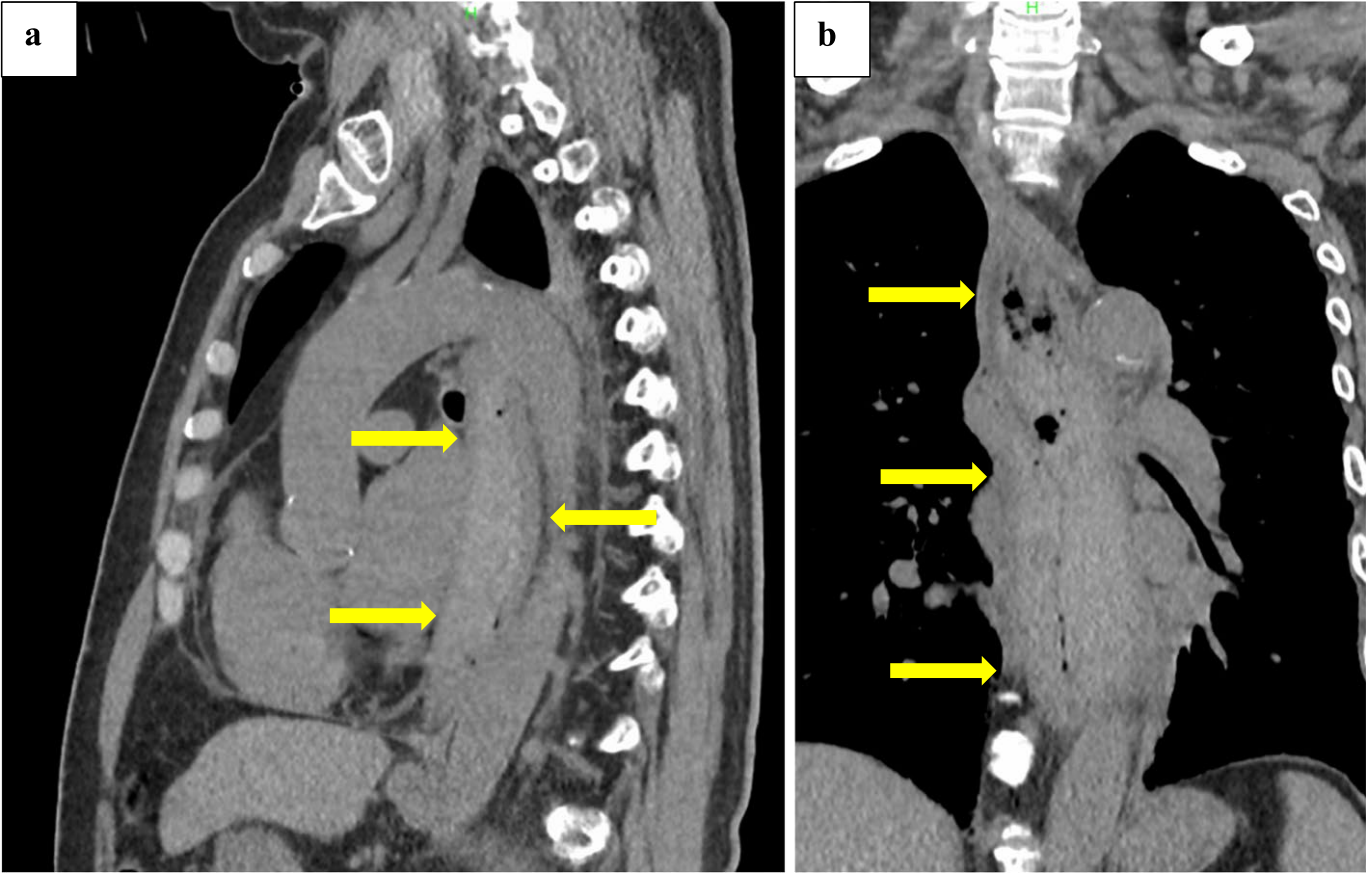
Plain CT on day 5 of illness. a Sagittal, b coronal. A high-density area due to hemorrhage is seen in the region of the wall thickening seen on CT on admission. Compared to the CT scan at the time of admission, it was thought that the bleeding was continuing
